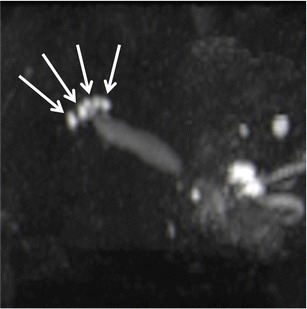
MRI of gallbladder adenomyomatosis: the pearl necklace sign. On heavily T2-weighted images, like in this maximum intensity projection reconstruction of a volumetric MRCP, a lot of RAS can be identified one next to the other around the gallbladder, leading to the so-called pearl necklace sign
